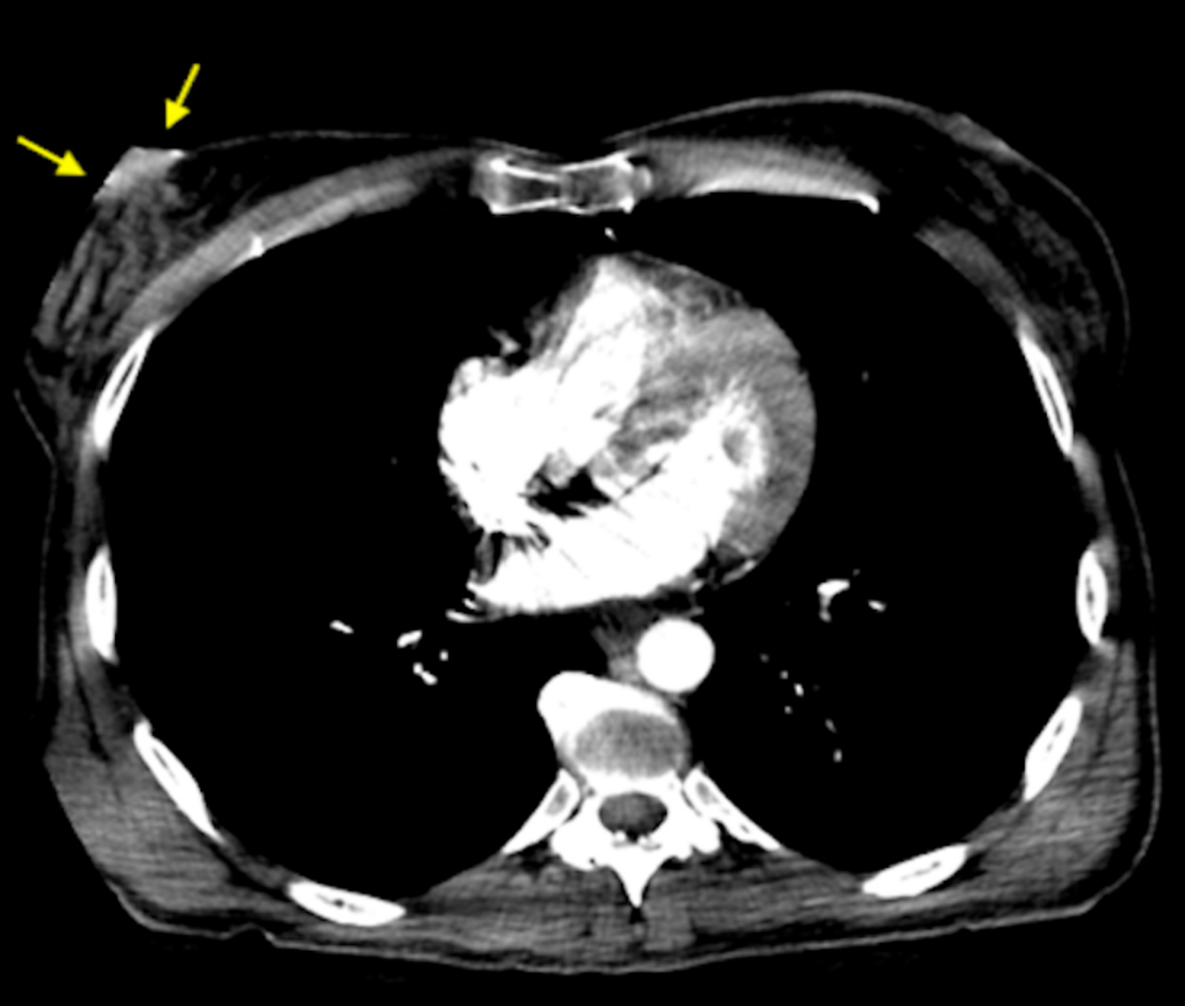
Axial image of a trauma protocol CT chest performed three years prior, in retrospect, shows asymmetric right nipple enhancement (yellow arrows), relative to the left nipple
